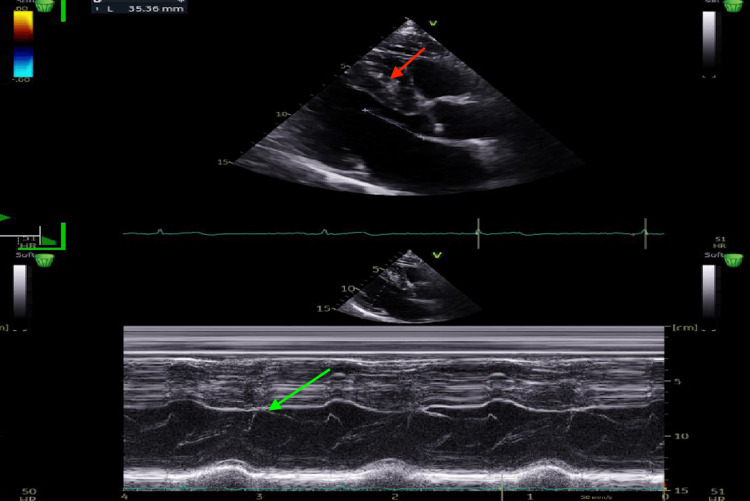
Parasternal Long-Axis M-Mode Transthoracic Echocardiography Parasternal long-axis M-mode transthoracic echocardiography demonstrating asymmetric septal hypertrophy (red arrow) and systolic anterior motion of the mitral valve (green arrow).
